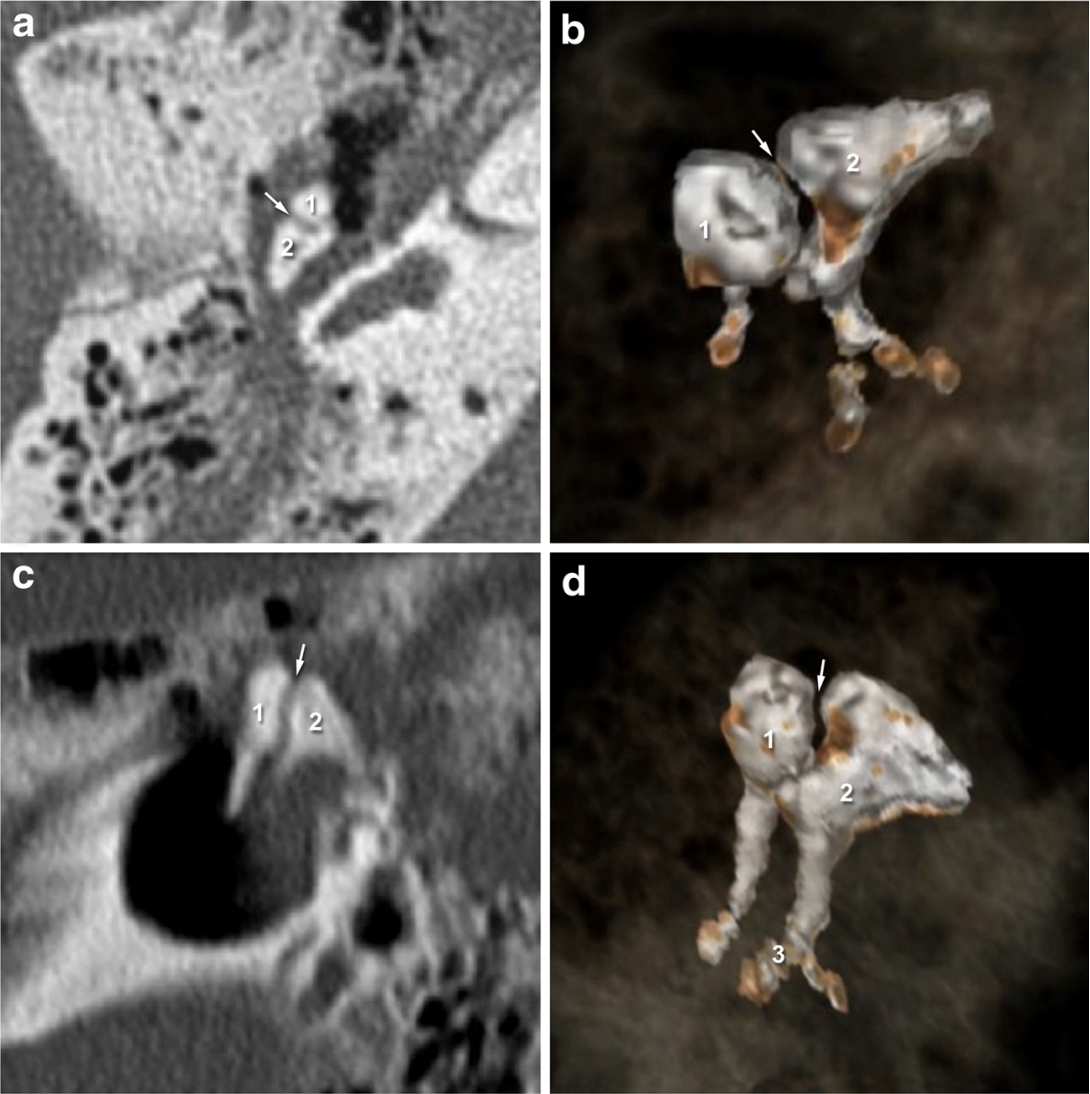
2-D-CT images (a and c) in the axial and coronal planes showing the incudo-malleolar joint after temporal bone trauma. The incus (2) is no longer in contact with the head of the malleus (1) on 2-D and 3-D-CT acquisition (b and d)
